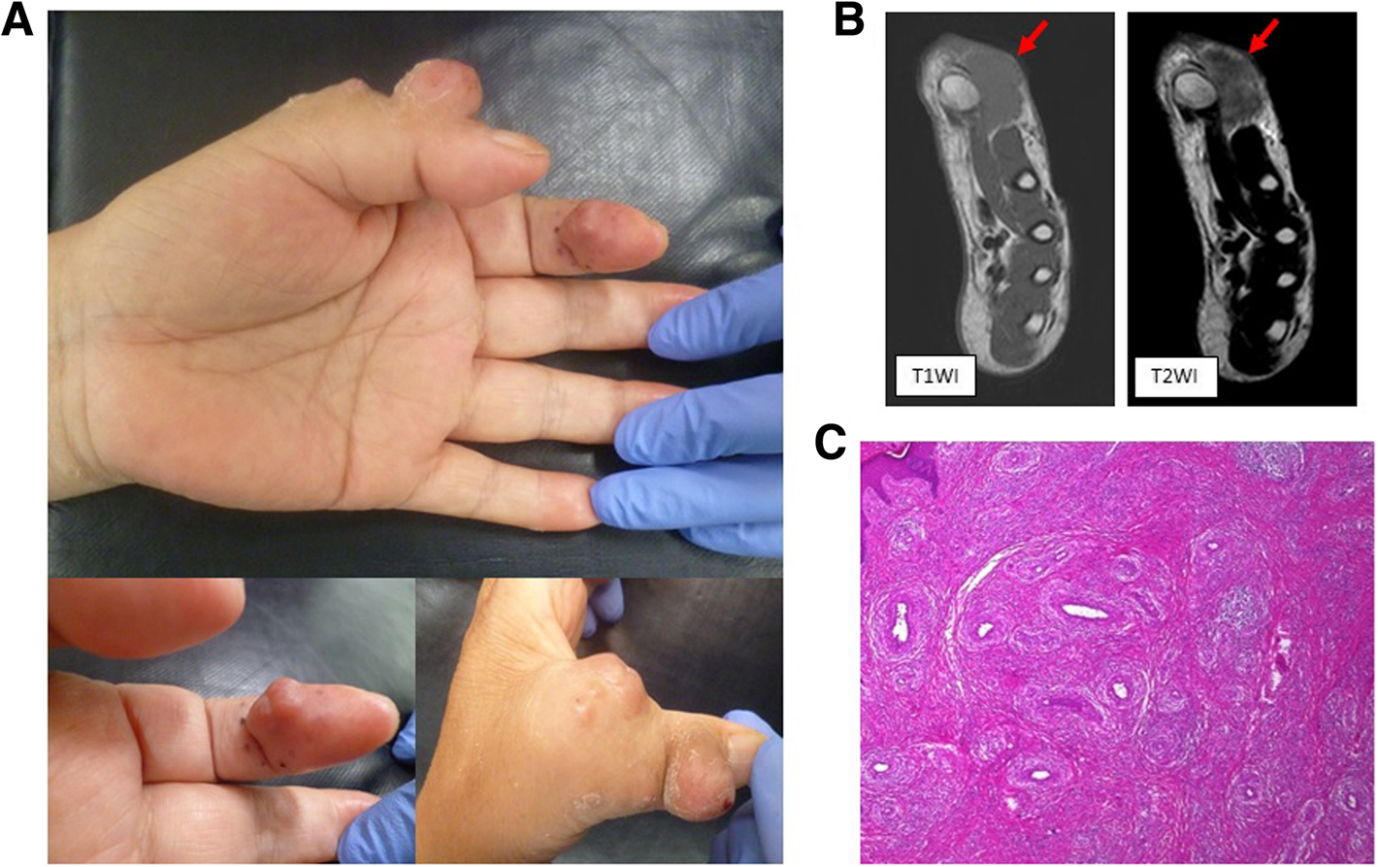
a Skin masses observed on the base of the left thumb and on the volar side of the index finger. b Magnetic resonance imaging of the lesion (red arrows) on the dorsal side of the thumb showed isointensity in T1-weighted images and high intensity in T2-weighted images. The tumor was confined to the skin. c Increase in the number of blood vessels in the dermal layer and eosinophilic infiltrates were noted around vascular endothelial cells. *T1WI* T1-weighted image, *T2WI* T2-weighted image
